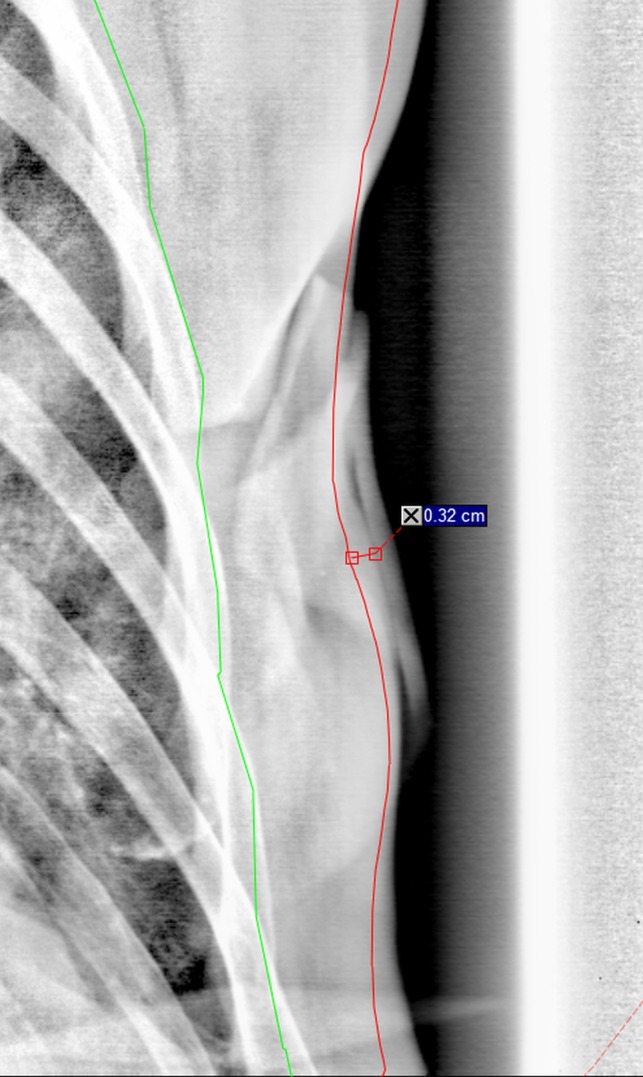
Measurement of tissue swelling or shrinkage d_s_ in the tangential image as the distance of the skin from the original CT‐based skin contour.
